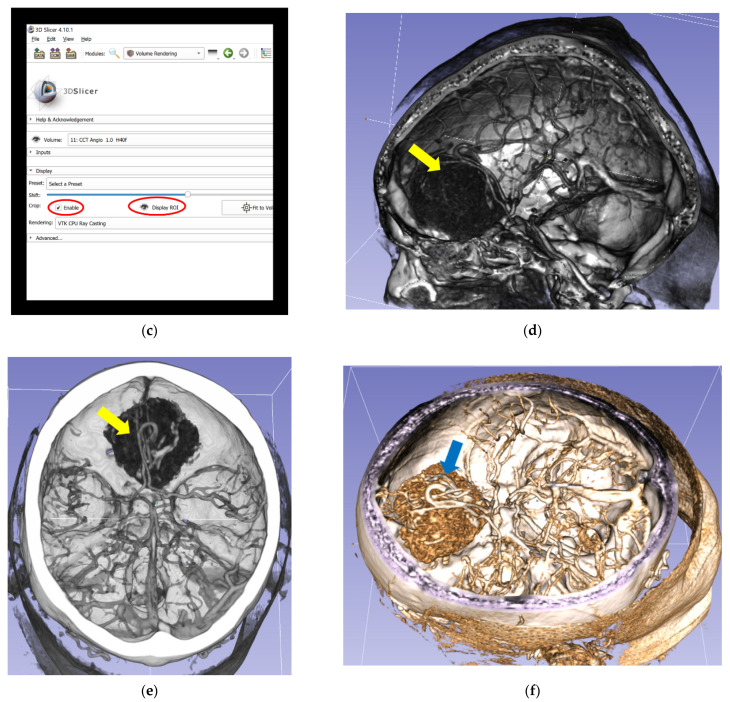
Reconstruction process of 3D-VR images from 2D-CT modalities (including CTA) and completion of the final VR scene in the 3D Slicer. (a) Import of original CT and CTA data in an anonymized DICOM format into 3D Slicer software to create a patient-specific database and selection of “CCT Angio-Default” (blue arrow) in the volume rendering window (red circled). (b) Performance of 3D-VR reconstruction of a skull (yellow arrow) in the volume rendering window. (c) Activation of the ROI function (red circled), which enables visualization of the meningioma and relevant vascular anatomy from different perspectives by partial omission of skull bones. (d) Lateral aspect of the meningioma (yellow arrow) and relevant vascular anatomy, simplified by using the ROI function. (e) Superior aspect of the meningioma (yellow arrow). (f) Superior lateral aspect of the meningioma (blue arrow) with different color displays. 2D, two-dimensional; 3D, three-dimensional; CT, computed tomography; CCT, cranial computed tomography; CTA, computed tomography angiography; DICOM, digital imaging and communications in medicine; ROI, regions of interest; VR, virtual reality.
